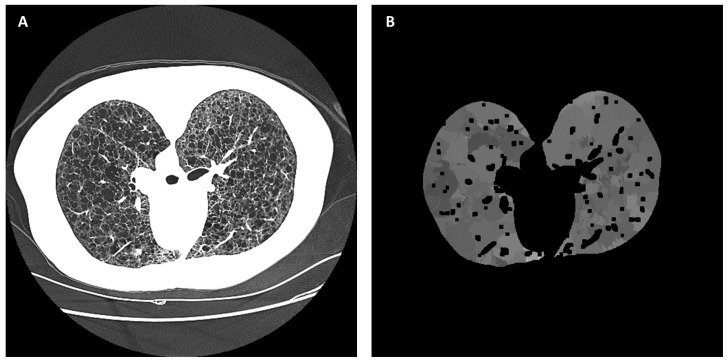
An example of deriving the radiodensity distribution of non-cystic parenchyma in the lungs by a local smoothed histogram filter. (A) The raw HRCT section is displayed on a grayscale of −1100 HU to −500 HU. (B) The calculated radiodensity distribution of non-cystic parenchyma in the lungs. The dark voids in the distribution are areas of bright vessels.
